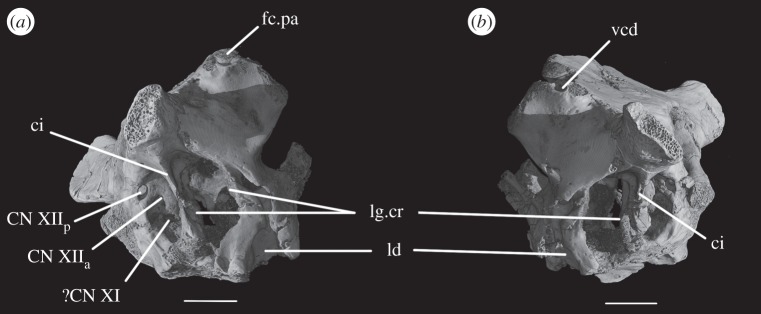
CT reconstructions of the braincase of SAM-PK-7696 (*a*) in right posterolateral and slightly ventral view and (*b*) in left posterolateral and slightly dorsal view. For abbreviations, see table 1.
